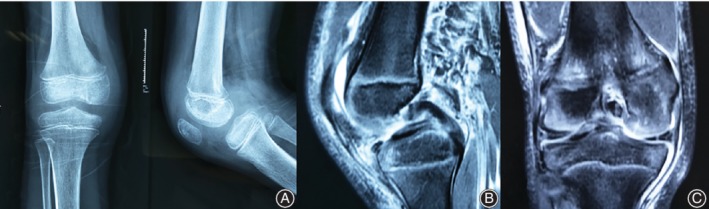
Preoperative X‐ray film showing a thin avulsed fragment of the posterior cruciate ligament arising from the femoral attachment (A). Preoperative MRI of the right knee showing an avulsion of the PCL at the femoral insertion (B) and a meniscus tear in the body of medial meniscus (C).
